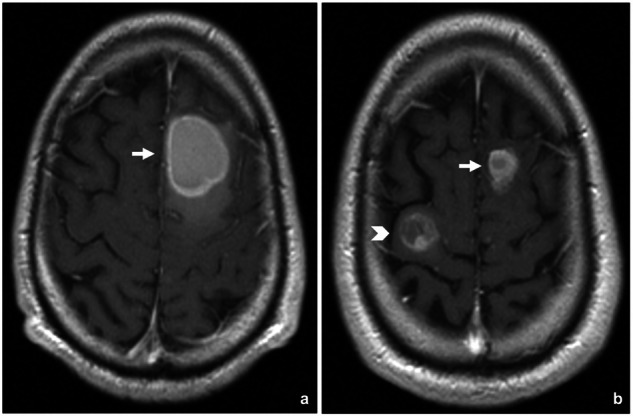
Dissociated response in a patient with brain metastases from melanoma treated with Ipilimumab and WBRT. In the baseline brain MRI (a), a left frontal metastasis is identified (arrow in a). In a subsequent follow-up scan during treatment 90 days after baseline (b), the initial lesion has responded to treatment, showing a significant reduction in size (arrow in b). However, a new lesion has appeared in the contralateral frontal lobe (arrowhead in b), indicating progressive disease and a dissociated response to treatment
